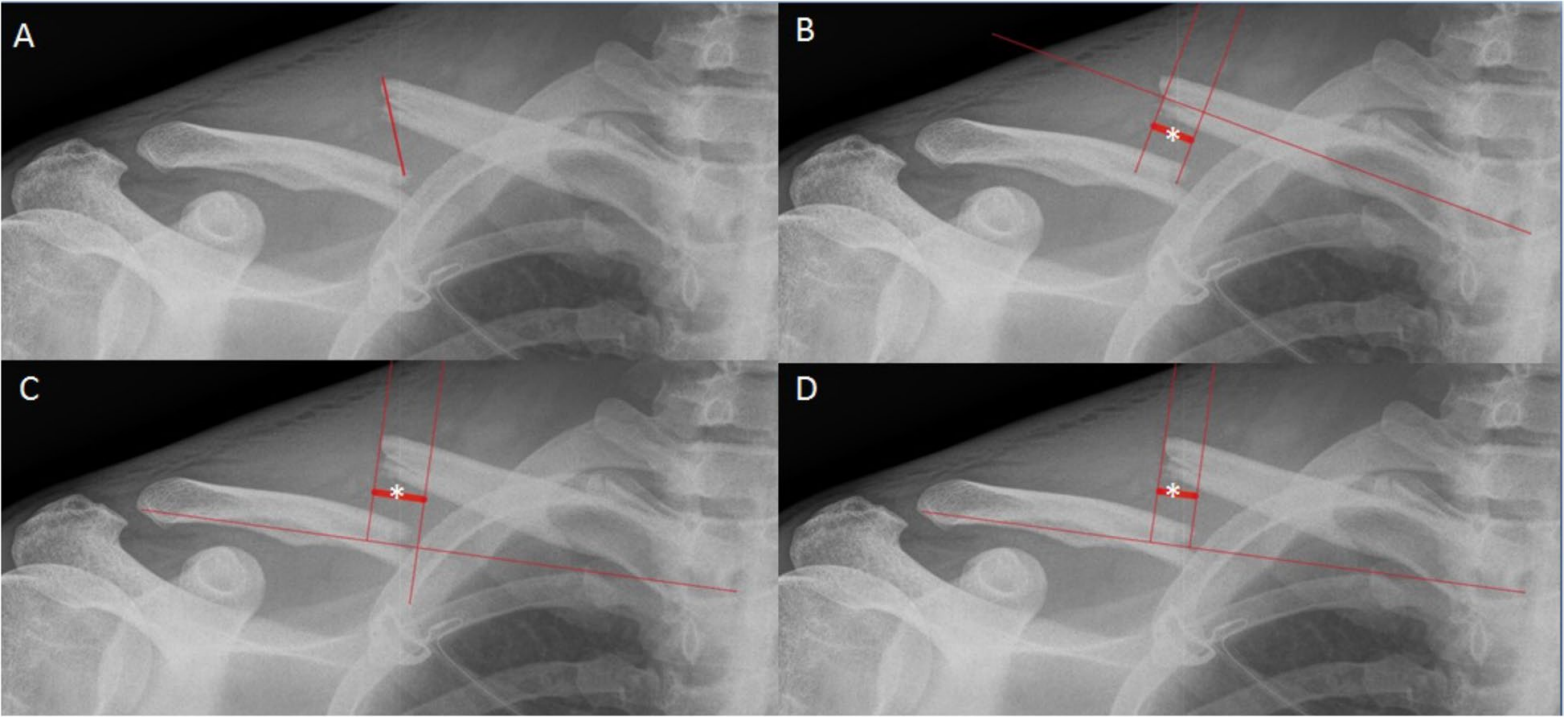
Radiographic midshaft clavicle fracture shortening measurement methods: A Jeray et al., B Silva et al., C Smekal et al. and D HUG method. See text for description
